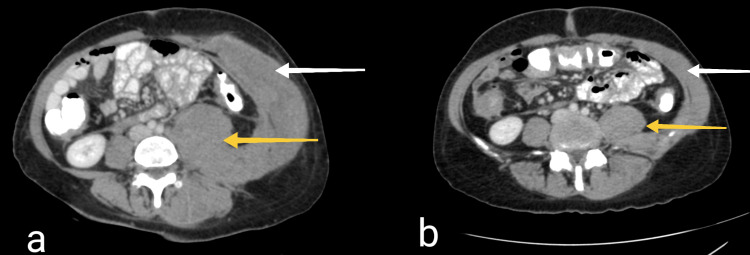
Contrast-enhanced abdominal computed tomography (a) The initially performed CT demonstrates diffuse thickening of the gastric wall, epiploic seeding, multisegmental serosal thickening of the gastrointestinal tract, as well as dramatic diffuse thickening of the muscles of the back (yellow arrow) and the anterolateral abdominal wall on the left side (white arrow). (b) The CT performed 16 months after the initial diagnosis, after the completion of chemotherapy, demonstrates a substantial improvement concerning primarily the abdominal wall invasion.
